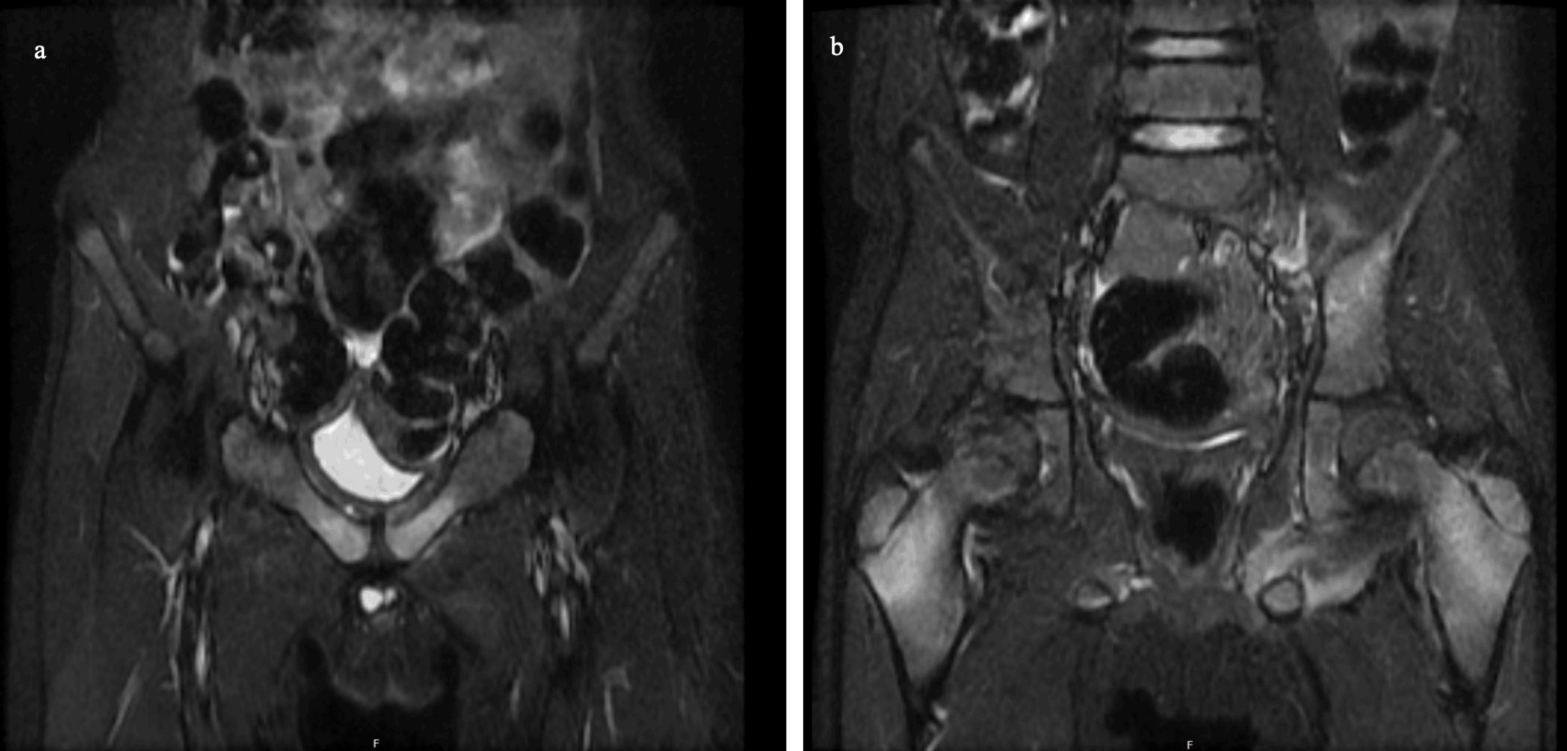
Pelvic MRI showing erosive findings at the left sacroiliac joint, asymmetric bone marrow edema at the bilateral pubic rami, and greater trochanteric apophysis/periphyseal region with adjacent intramuscular edema and periostitis
